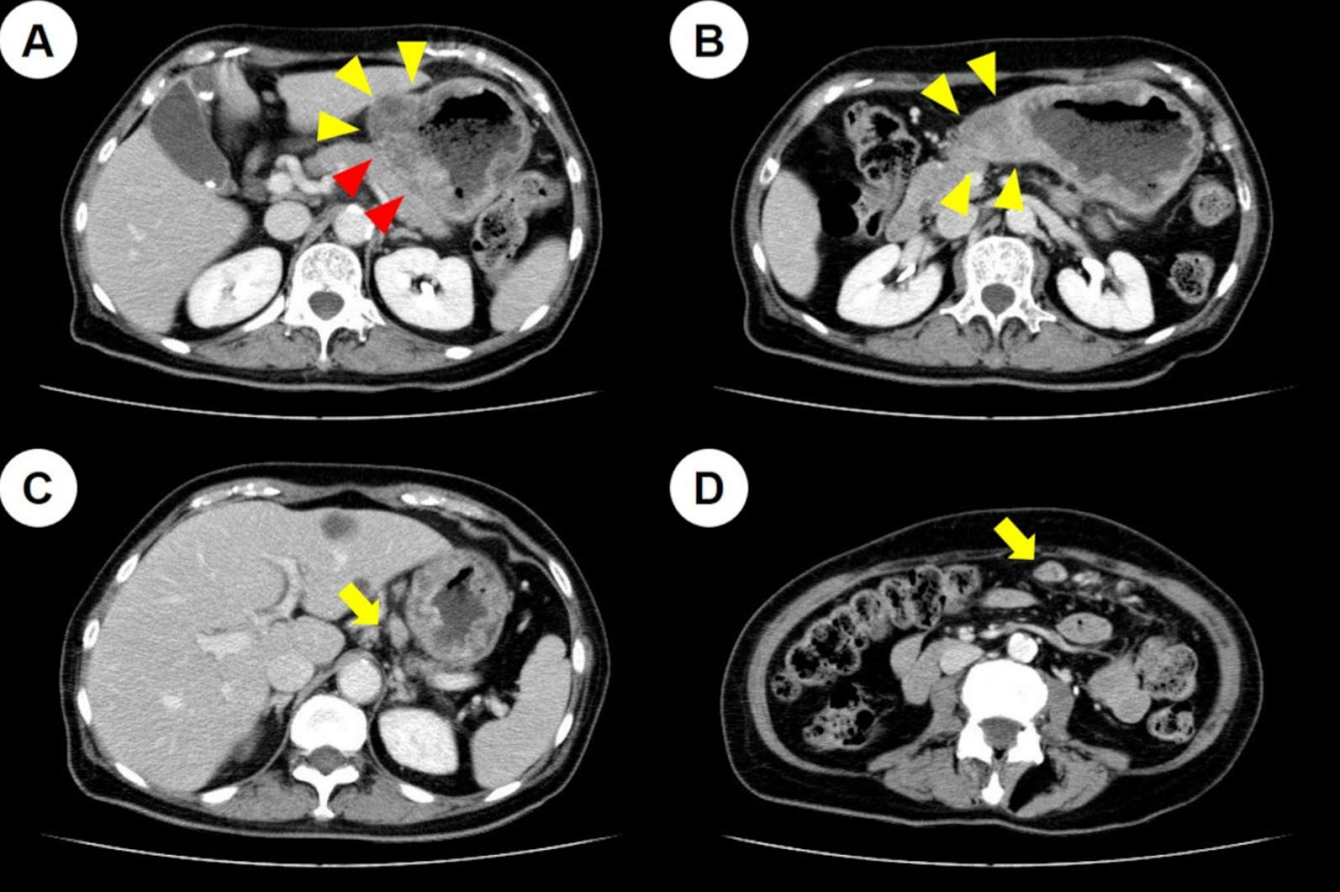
Computed tomography findings before chemotherapy. A Wall thickening in the lower part of the gastric body (yellow arrowheads) and unclear boundaries between the tumor and the pancreas (red arrowheads) were demonstrated. B Full-circumferential wall thickening in the lower part of the gastric body was observed (yellow arrowheads). C Enlarged lymph node of lesser curvature was observed (yellow arrow). D Enlarged lymph node of greater curvature was observed (yellow arrow)
